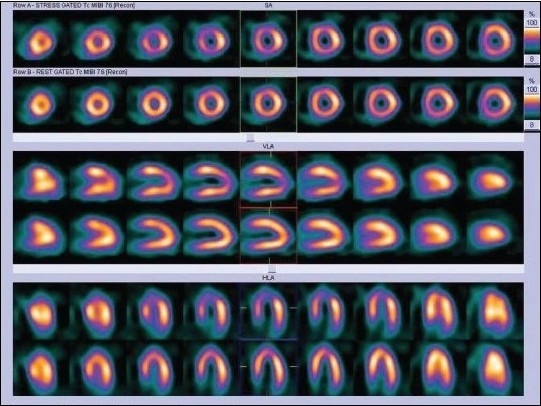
Same day stress - rest MIBI SPECT images (displayed in two different color scales) of a 12-year-old patient with Kawasaki disease showing mild reversible ischemia in the apex, anteroapical, and septal segments of the LV
